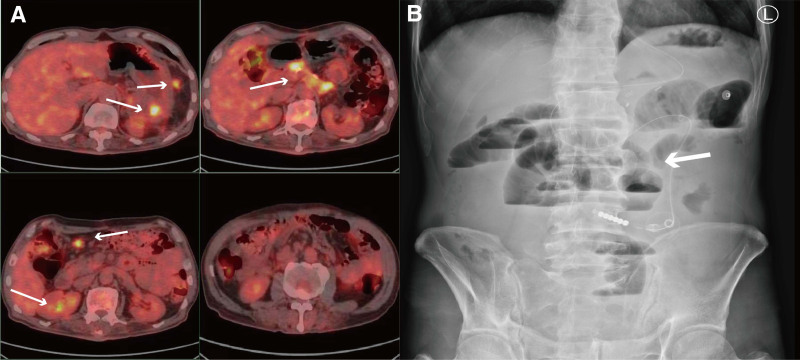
The imageological examination of case 2 patient. (A) The PET/CT (October 4, 2023) showed soft tissue thickening at the surgical site of pancreatic cancer, suggesting invasion of the adjacent gastric wall with increased FDG metabolism. (B) The CT scan (taken on November 8, 2023) of the abdomen further showed small bowel obstruction and fluid in the abdominal cavity. CT = computed tomography.
